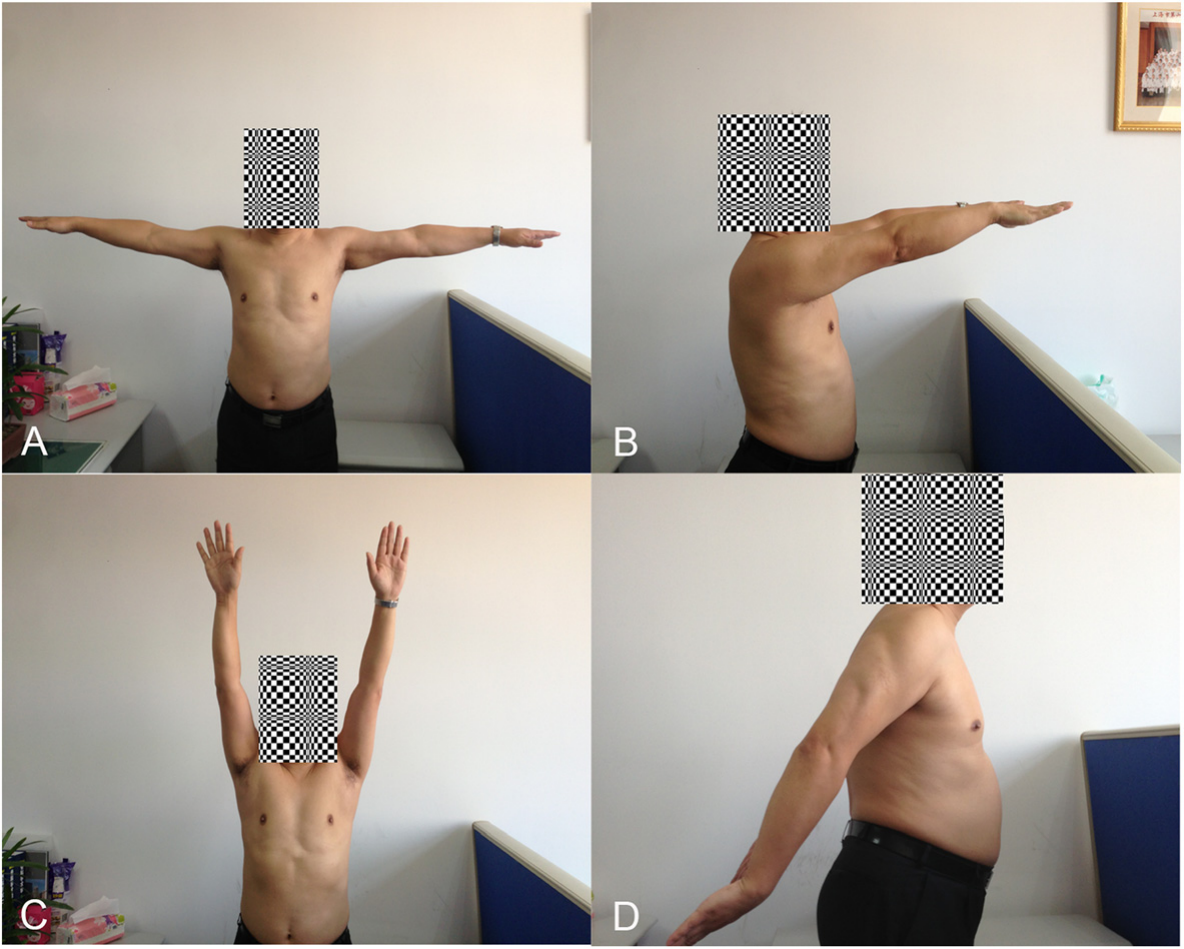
Functional results of the shoulder joint. The patient with radiographic data showed in Fig. 1 had excellent functional outcome. The involved shoulder joint had the same range of motion as the contralateral in abduction (a), anterior extension (b), elevation (c) and posterior extension (d). (The patient has provided consent to appear in the Figure)
